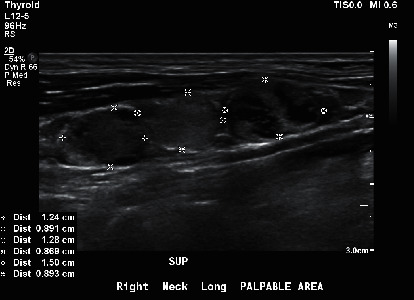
Ultrasound transverse image of lymphadenopathy in the right cervical area with distortion of normal morphology and cystic necrosis.
